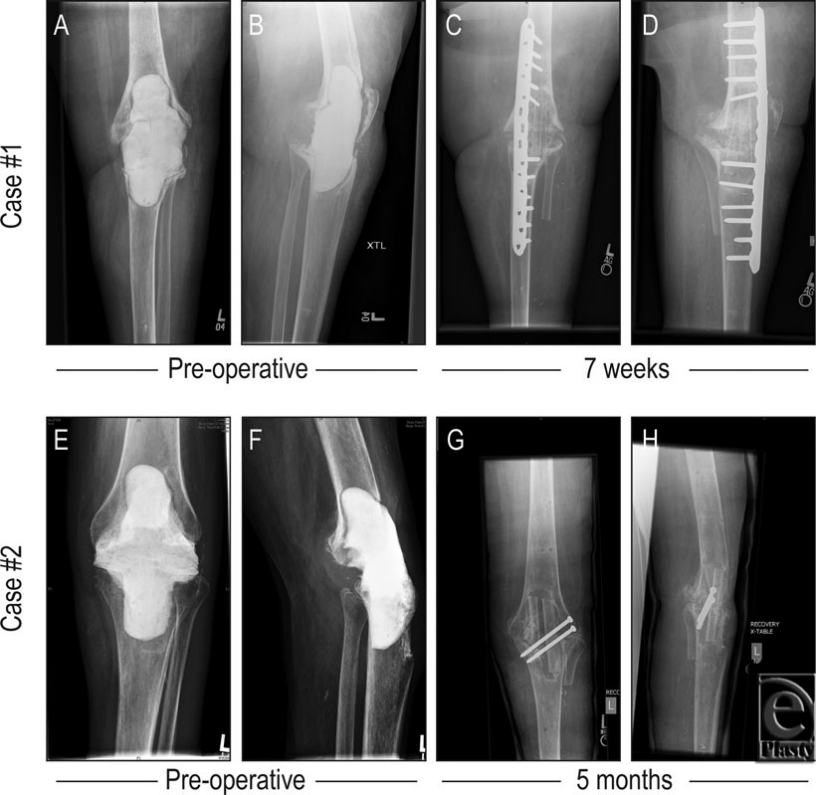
Radiographic documentation of cases. (*a*) Preoperative AP roentogram of infected knee joint of case 1. (*b*) Preoperative lateral roentogram of infected knee joint of case 1. (*c*) Postoperative AP. (*d*) lateral roentogram of case 1 at 7 weeks. (*e*) Preoperative AP roentogram of infected knee joint of case 2. (*f*) Preoperative lateral roentogram of infected knee joint of case 2. (*g*) Postoperative AP. (*h*) lateral roentogram of case 2 at 5 months.
